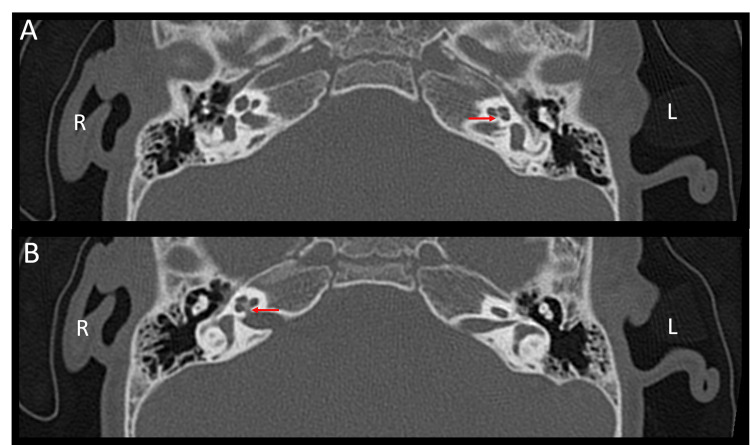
Axial CT images of the IAC. A) The left (L) bony cochlear nerve canal with apparent stenosis (red arrow). B) The right (R) bony cochlear nerve canal (red arrow) is visualized in the image. Image A was selected as the better plane for visualizing the left bony cochlear nerve canal, while image B was chosen to best demonstrate the right bony cochlear nerve canal, as both could not be adequately visualized in a single plane. CT: computed tomography; IAC: internal auditory canal; R: right side; L: left side.
